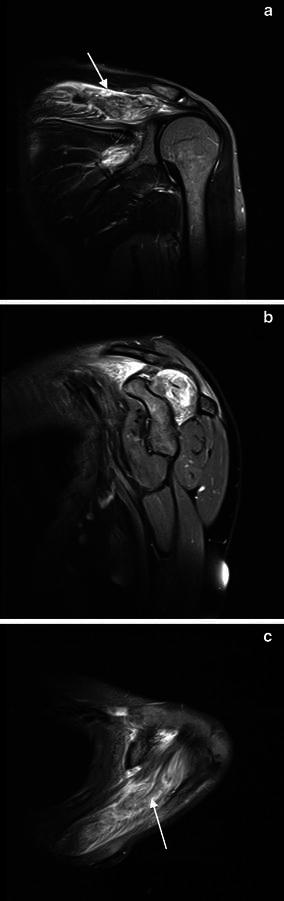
Magnetic resonance imaging performed 1 day after trauma. a T1 short-tau inversion-recovery-weighted coronal view shows disruption of the musculotendinous junction of the supraspinatus with hematoma and edema involving the entire muscle belly; the intramuscular septum is inhomogeneous and slackened (*arrow*). b Proton density fat-saturated sagittal imaging at the level of the scapular Y view shows a intramuscular hematoma in the posterior portion of the muscle belly and perimuscular edema. c The intramuscular septum is clearly slackened on proton-density fat-saturated axial view (*arrow*)
